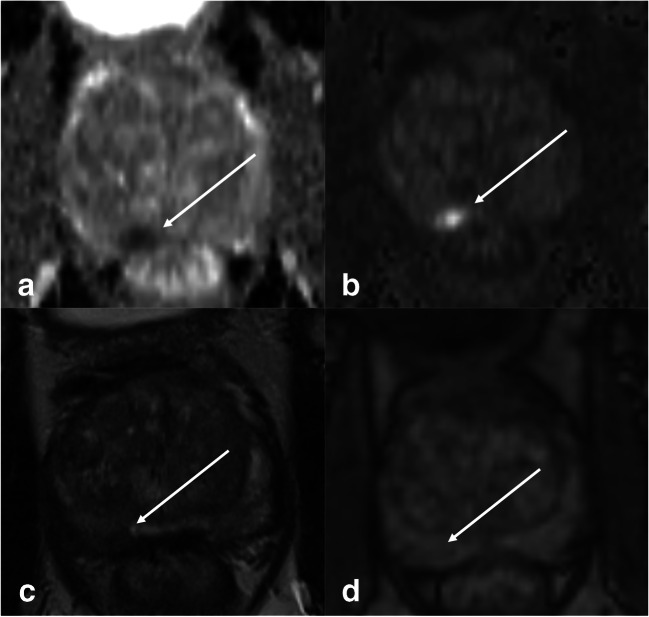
72-year-old man with a biopsy-proven ISUP 2 invasive prostate cancer visible on multiparametric MRI (white arrows). The lesion measures < 15 mm and shows typical PI-RADS 4 features on DWI (a ADC map, b high *b*-value); the visibility is excellent. On T2w (c), lesion visibility is acceptable while on early DCE (d), the lesion presents with poor visibility and would not have been detected
